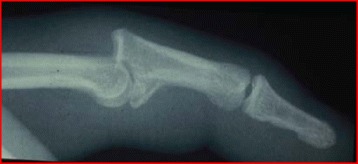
Lateral radiograph of a dorsal fracture dislocation of the PIP joint with 50 % articular involvement of the middle phalanx
